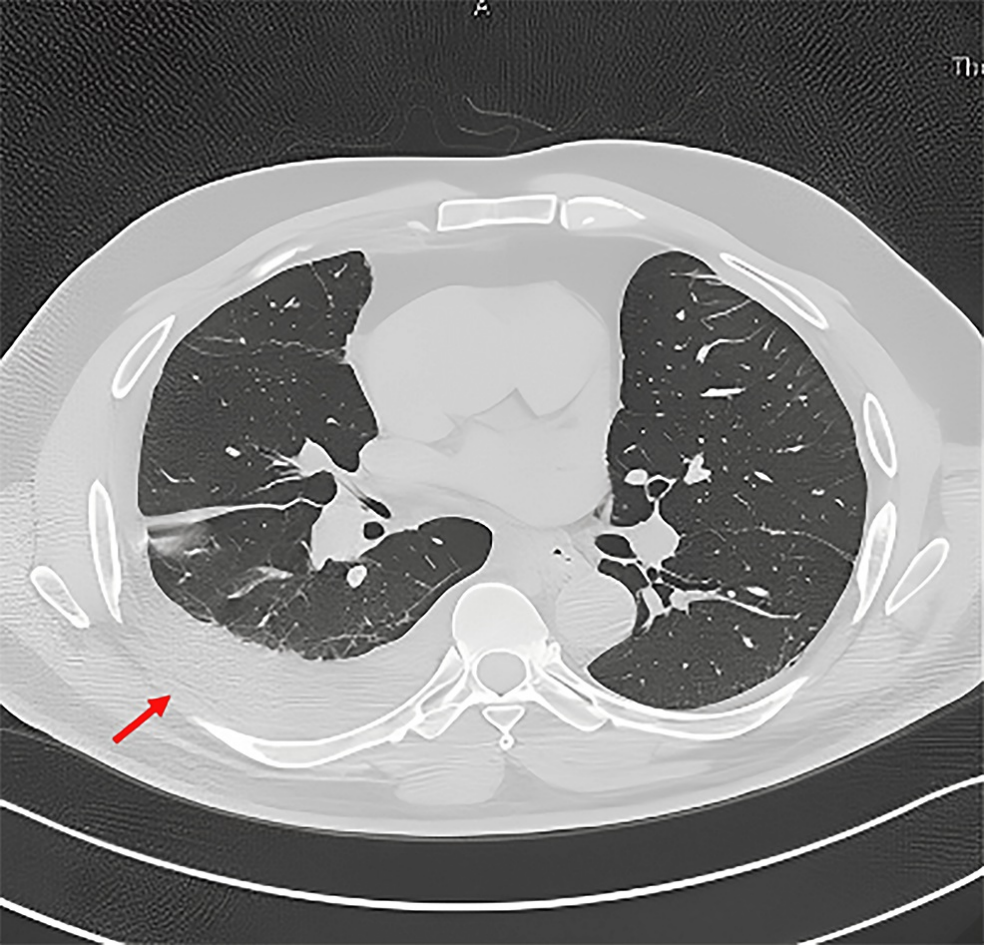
CT thorax showing a mild right-sided pleural effusion with lower lung segmental collapse
